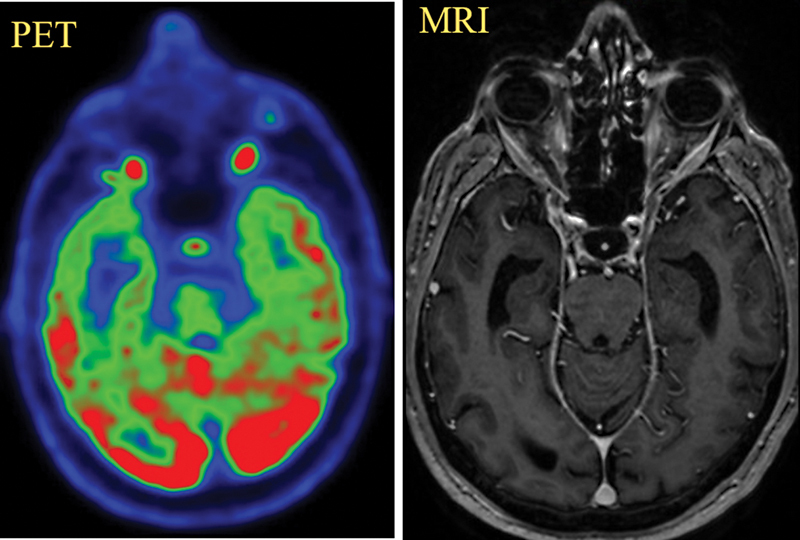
Positron emission tomography (PET) and magnetic resonance imagings (MRIs) of a 71-year-old male, with the case of acral melanoma; 18F-2 fluoro-2 deoxy D glucose PET/computed tomography scan was done for staging. Intermediate to high uptake is seen in normal pituitary gland.
